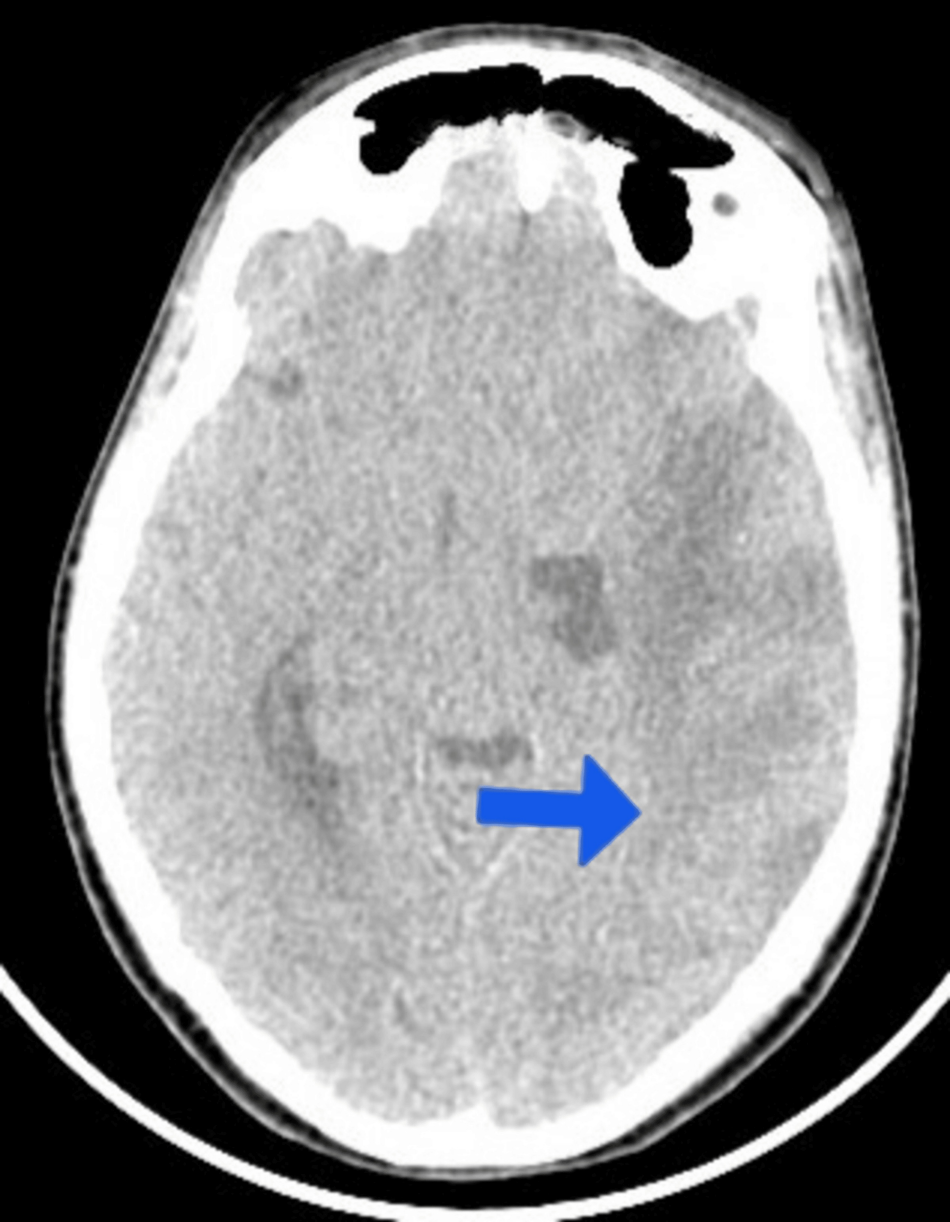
CT brain without contrast-axial view; the blue arrow shows hypodensity with surrounding edema in the left parieto-occipital region
